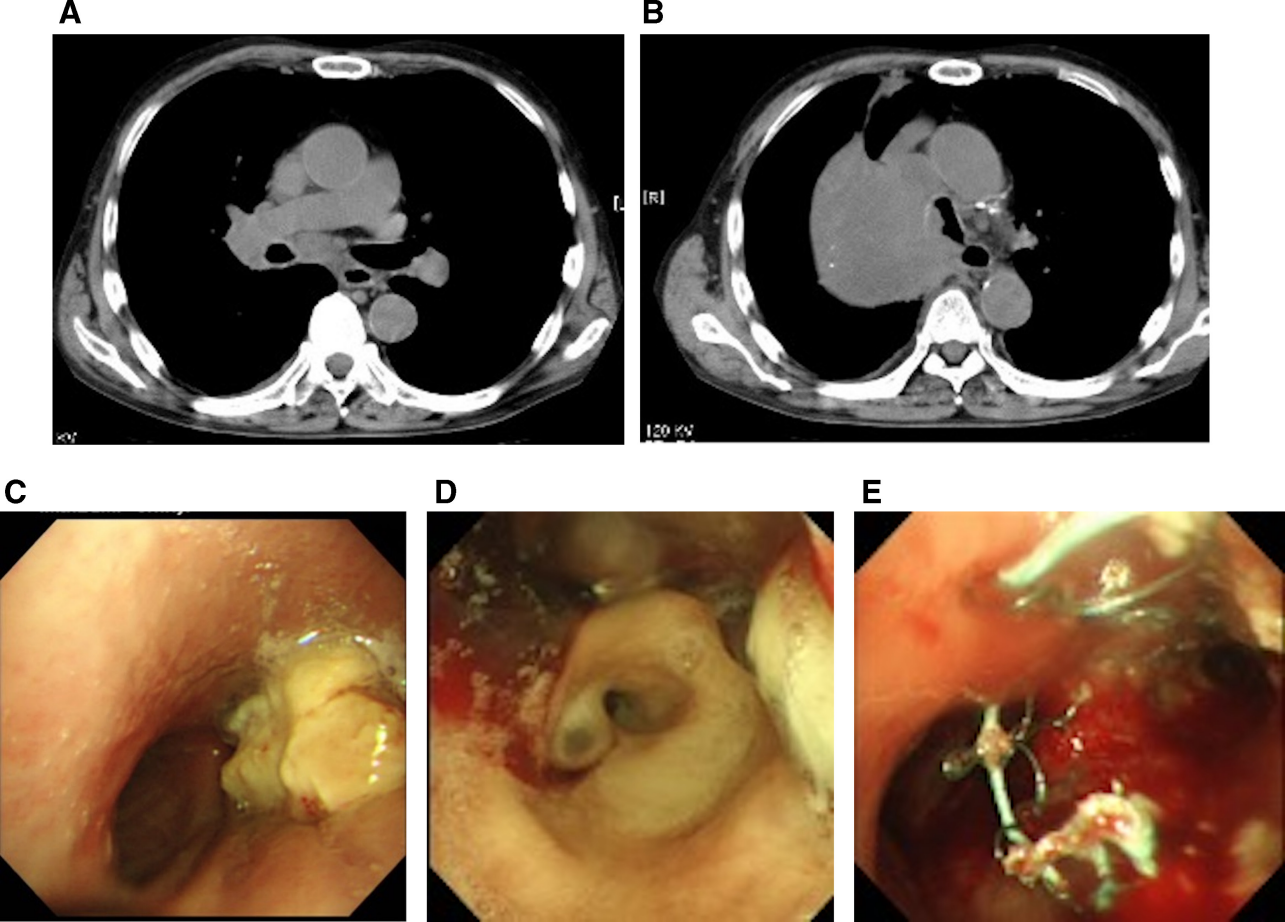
(A, B) Thoracic computed tomography demonstrated extrinsic compression of the trachea and complete obstruction extending from the right main bronchus to the right intermediate bronchus. (C–E) Bronchoscopic findings revealed that the trachea was compressed extrinsically, and the right main bronchus was directly obstructed by the tumour. An uncovered Ultraflex SEMS (14 × 60 mm) was placed in the right main bronchus, and a covered Ultraflex SEMS (14 × 60 mm) was deployed in the trachea.
